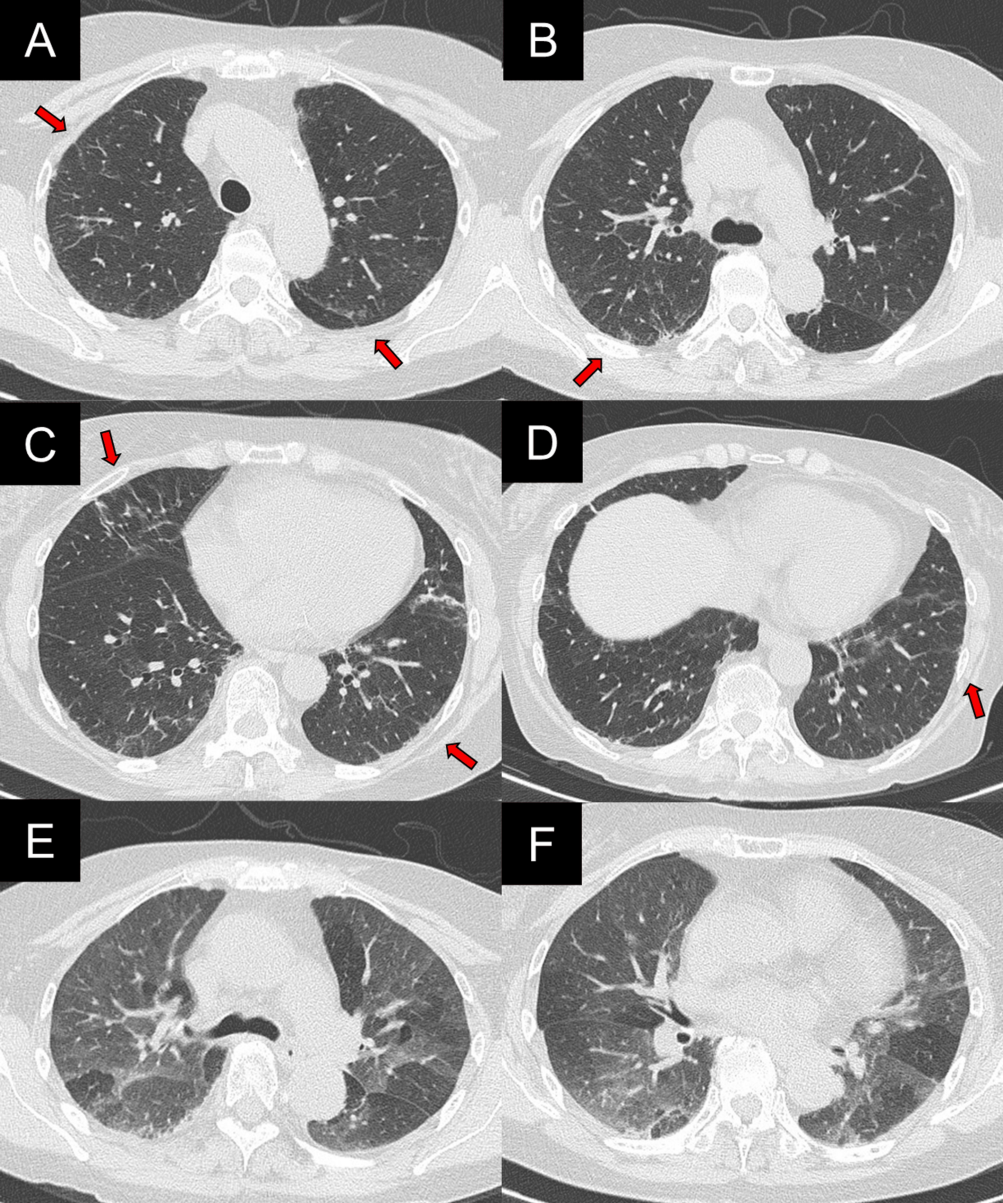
High-resolution computed tomography (HRCT) obtained two months after combination therapy (A-D) Inspiratory phase images showing improvement of GGOs (red arrows). (E-F) Expiratory phase images.
